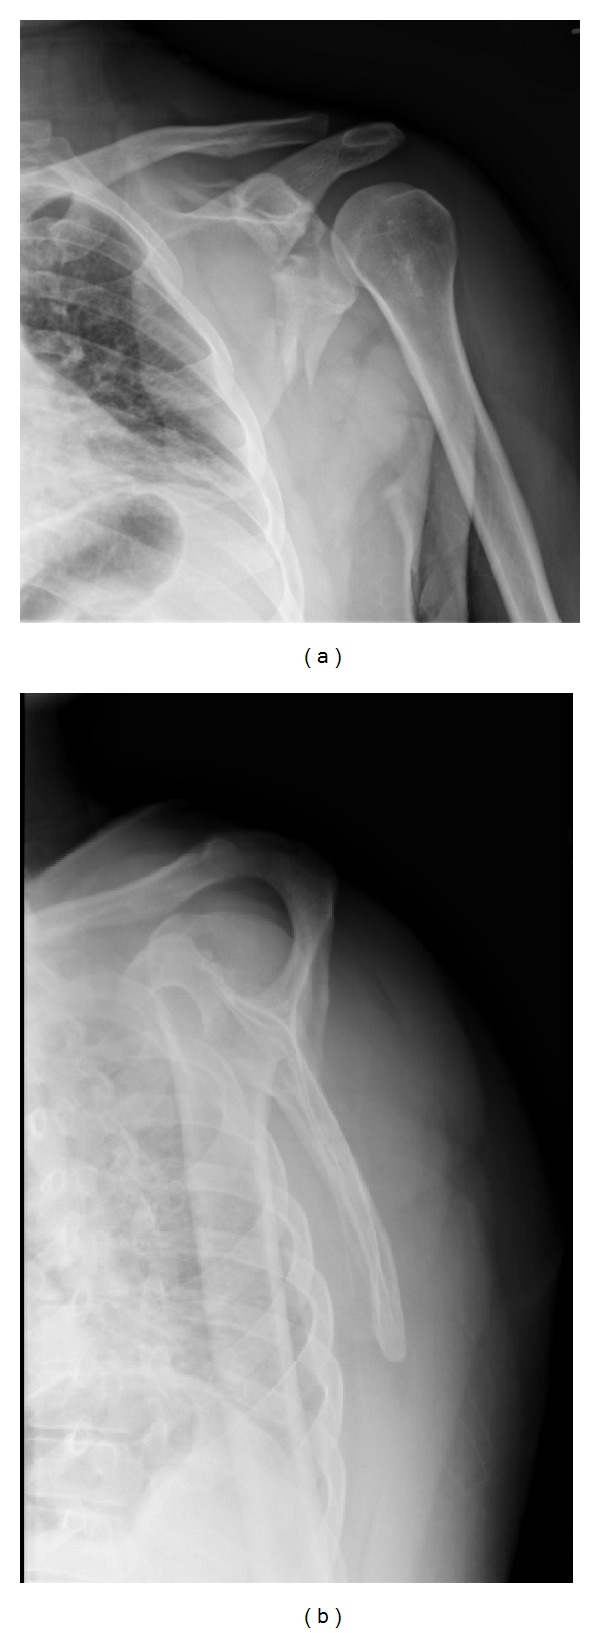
Anteroposterior (a) and lateral (b) radiographs of the left shoulder demonstrating a comminuted fracture of the lateral aspect of the left scapula with glenoid involvement.
